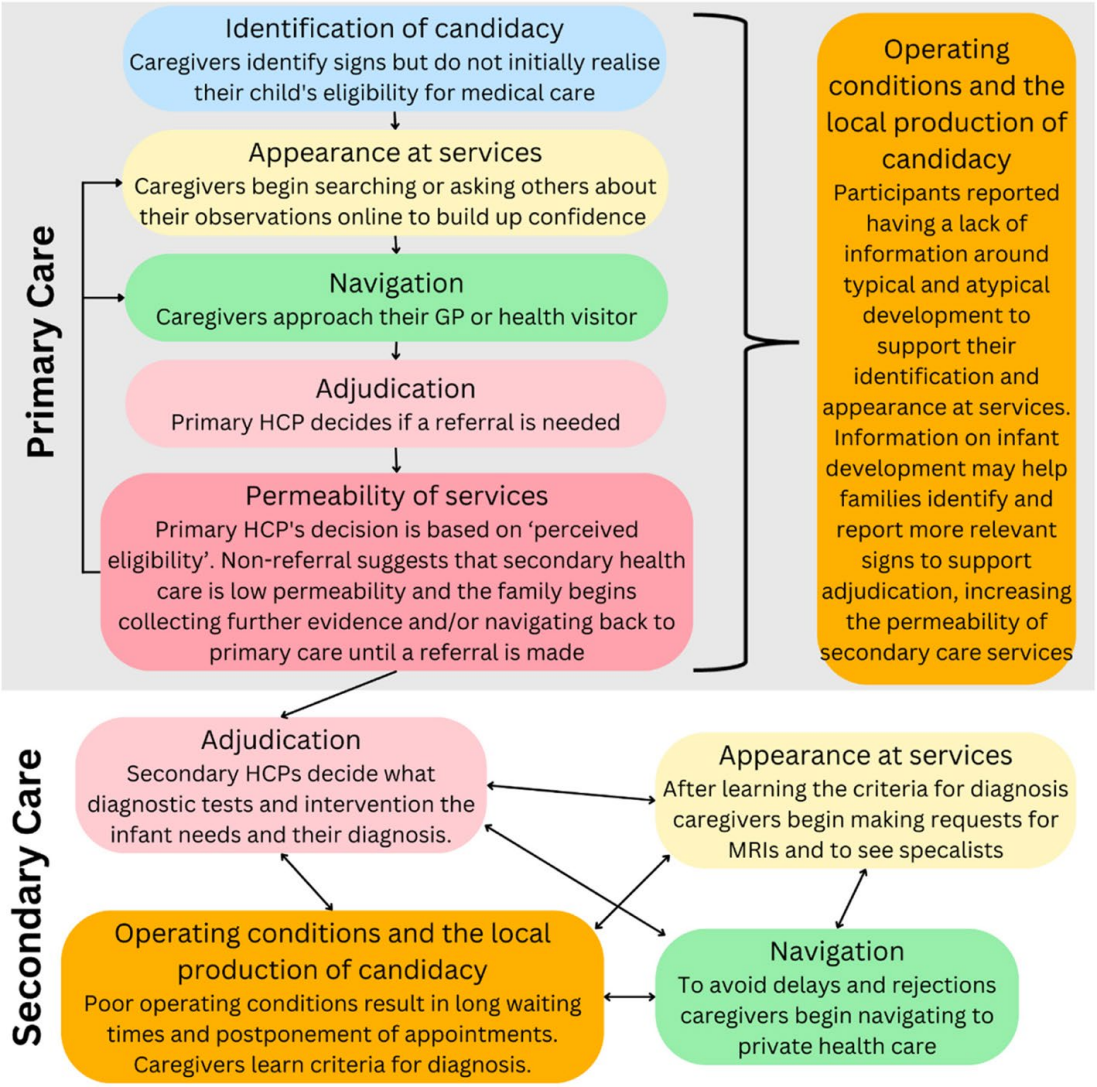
The order in which families of infants identified in the community with emerging motor problems and later diagnosed with CP go through the seven stages of candidacy. Candidacy is a dynamic process theory that describes how an individual negotiates their eligibility for health care with themselves and others. The dynamic nature of the theory means that the stages do not occur in a linear order, that two or more stages may occur at once, and that stages may reoccur. HCP – Health Care Professional. MRI – Magnetic Resonance Imaging brain scans
